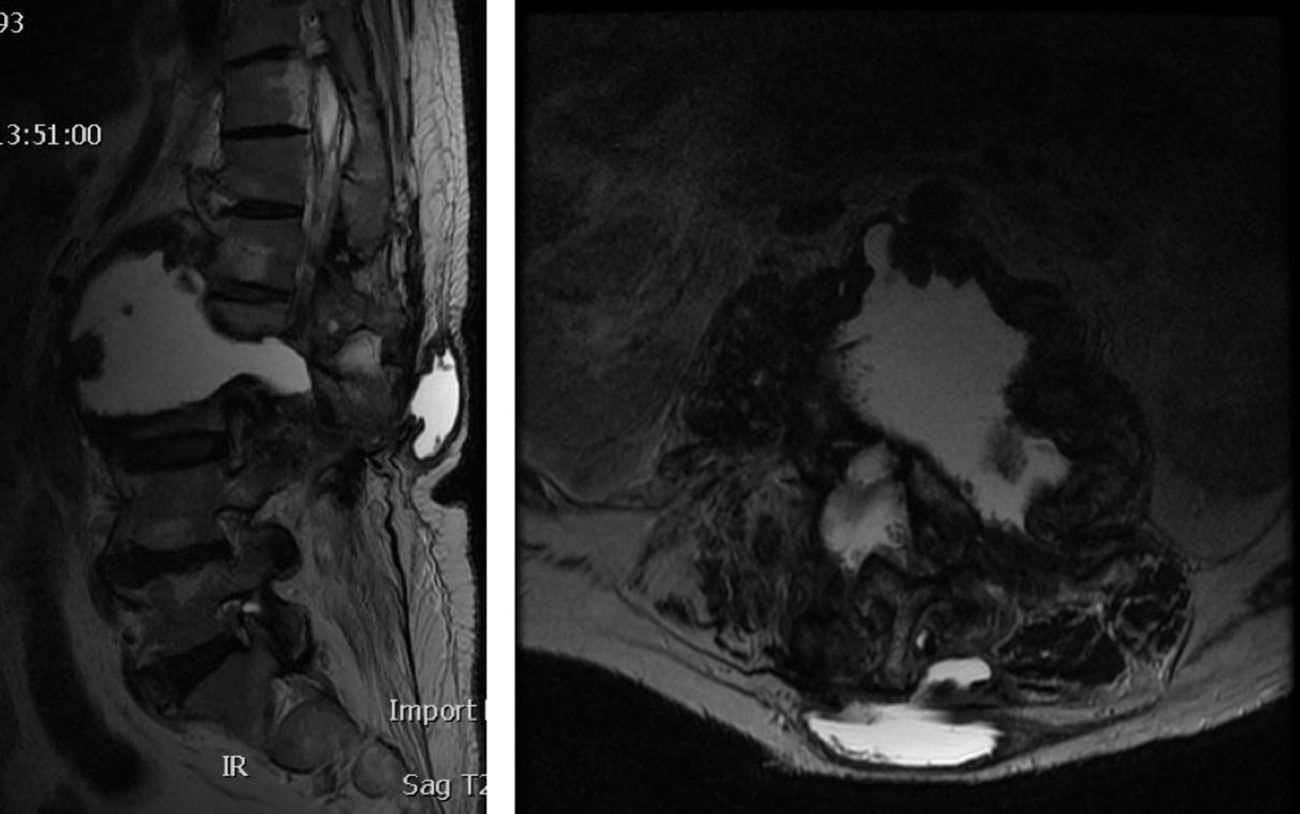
Pre-operative MRI in supine position showing large fluid filled cavitary lesion between L2 and L3 vertebrae extending to the posterior elements and associated bony resorption.
